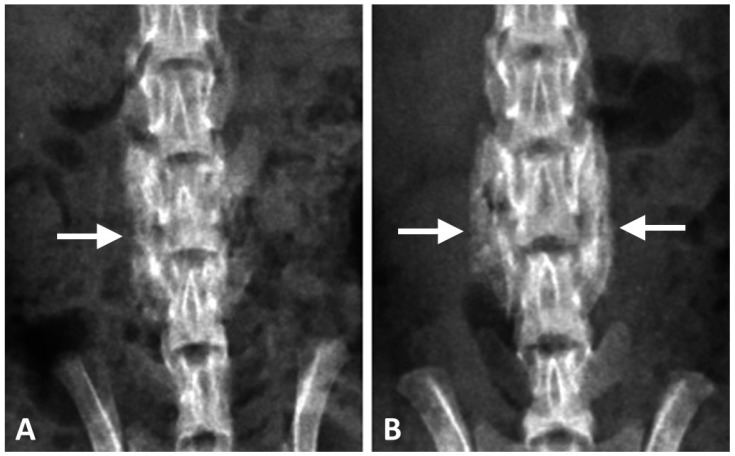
Posteroanterior radiographs after post-operative four weeks of the spines that underwent posterolateral lumbar fusion with (A) DCP-rich CPC or (B) DCP-rich CPC + 0.4 mL collagen. The arrows indicate fusion mass.
